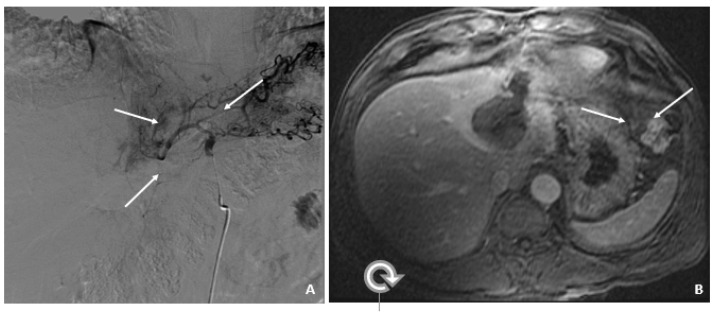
Transarterial Chemoembolization (December 2009). (A) The selective left hepatic artery injection shows a blush of contrast corresponding to the hypervascular tumor (arrows). (B) An axial T1-weighted fat-suppressed portal-phase postcontrast MRI image 4 weeks following TACE shows lack of enhancement in the treated lesion with a small adjacent focus of necrosis (arrows).
